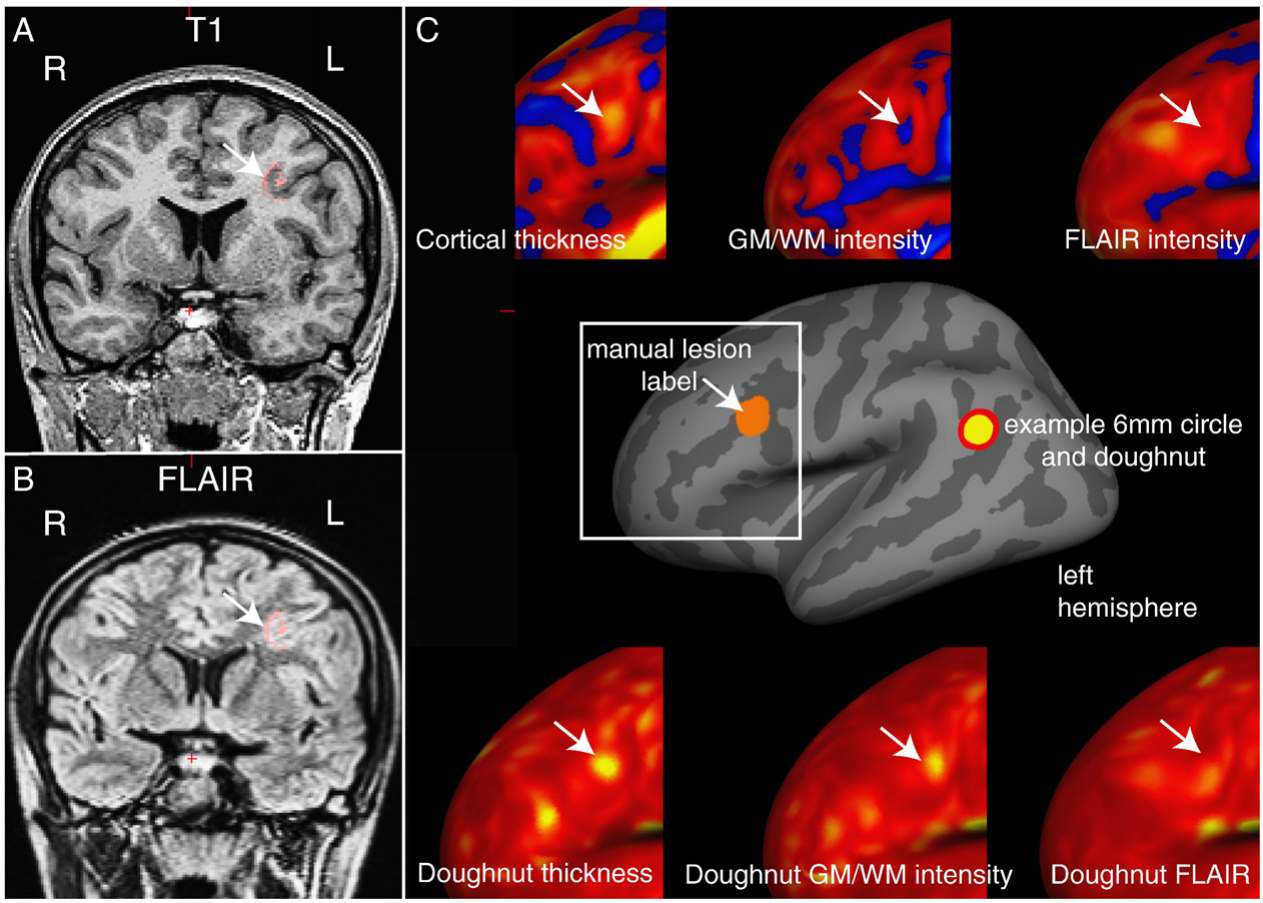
Example of “doughnut” method maps in a patient with a left middle frontal sulcus FCD. A) T1 image B) FLAIR image - manual lesion label in pink, white arrow indicates lesion. C) Inflated surface view with manual lesion label (orange) and example of 6 mm doughnut and circle. Upper panel – intra-subject normalised cortical thickness, grey-white matter contrast and FLAIR intensity (sampled at 50% cortical thickness) overlays around lesion area (white square). Lower panel – “doughnut” thickness, “doughnut” grey-white matter intensity and “doughnut” FLAIR (sampled at 50% cortical thickness). This lesion is characterised by a subtle increase in cortical thickness, though much less thick than the insula (bright yellow), subtle decrease in contrast at the grey-white matter boundary and no obvious FLAIR hyperintensity. “doughnut” thickness and “doughnut” grey-white matter intensity contrast highlight lesion, in this particular example “doughnut” FLAIR is of less use. All surface measures also identify other areas of cortex with extreme values and must therefore be used in combination.
